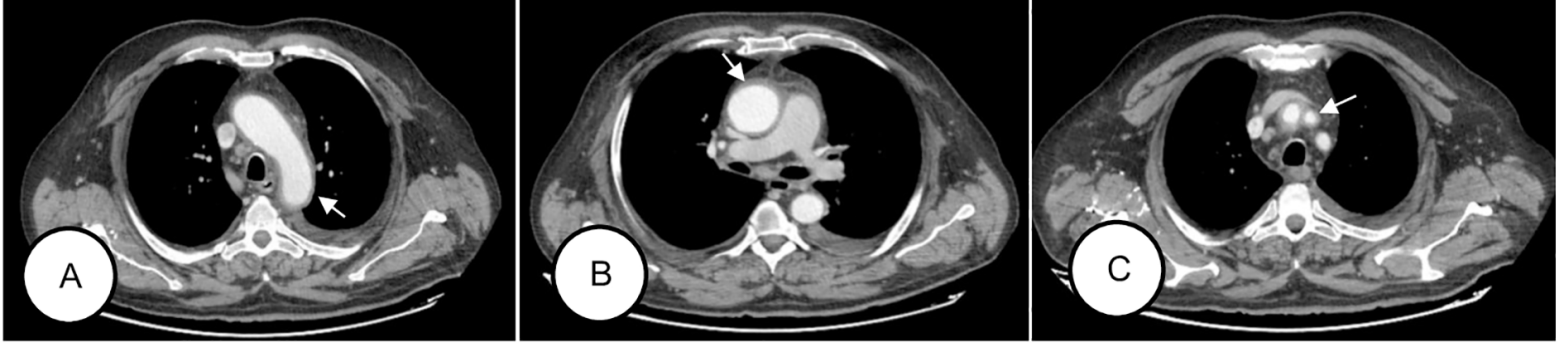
Anatomical extent of vasculitis (CT scan, November 2022, from left to right). (A) Involvement of the aortic arch. (B) Involvement of the ascending and descending thoracic aorta. (C) Involvement of the brachiocephalic trunk, right subclavian artery, origin of the left subclavian artery, and proximal segment of the right common carotid artery. GE Revolution 256-slice CT scanner of the chest, abdomen, and pelvis with biphasic arterial and venous injection of Ultravist iodinated contrast (300 mg I/ml) at a flow rate of 3 ml/s in an antecubital vein was used in the oncological follow-up study where aortic dissection was diagnosed.
